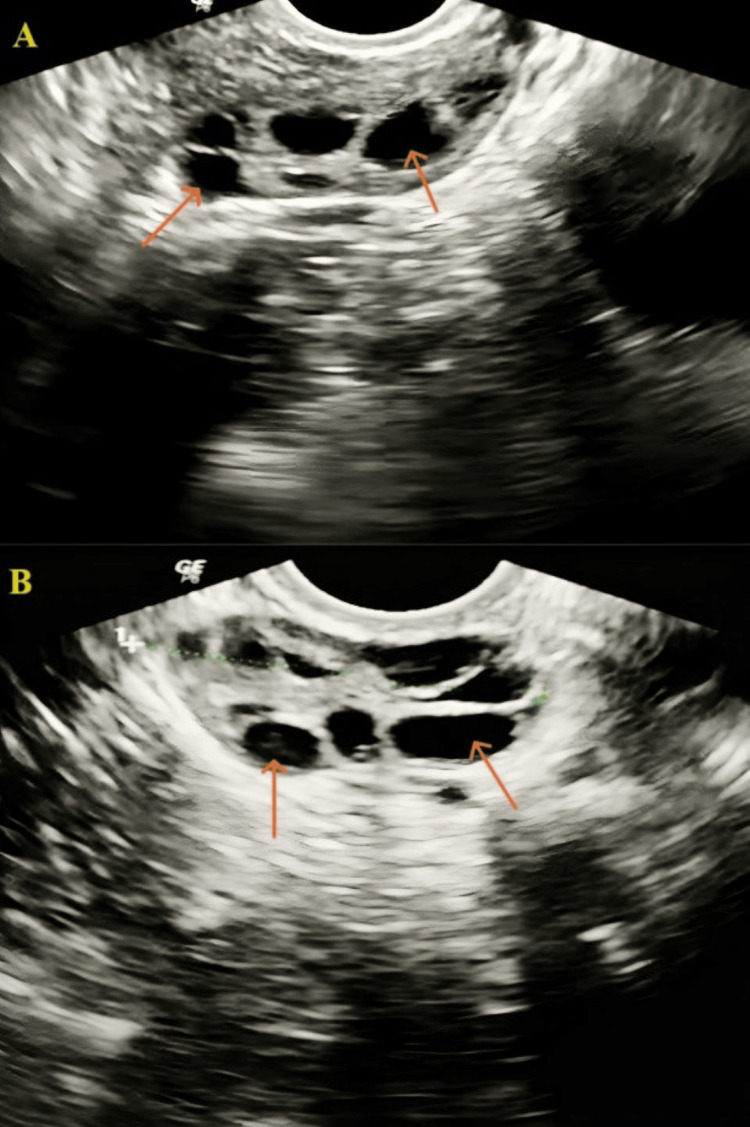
Transvaginal sonography image of the patient A: right ovary, B: left ovary. The orange arrow shows the ovarian cyst.
